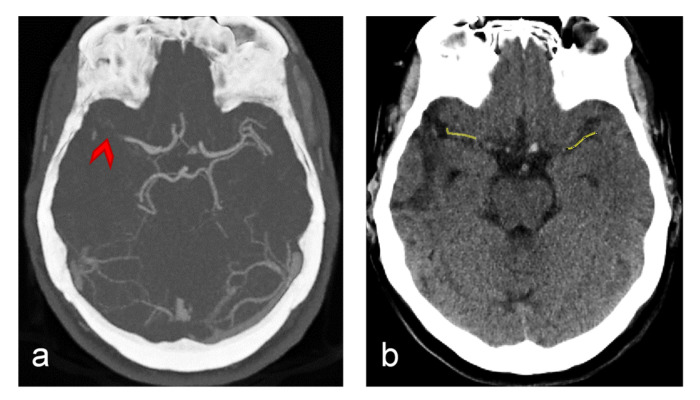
Representative brain CT images of an ischemic stroke patient in a slice with the middle cerebral (MCA) artery: (a) CT angiograph (CTA) image and (b) non-contrasted CT (NCT) image of the same slice. Red arrow in the CTA image points to the occlusion (thrombus), while yellow lines in the NCT image correspond to the lines along which HU values were measured in the occluded site (left) and in the symmetrical positioned non-occluded site (right).
